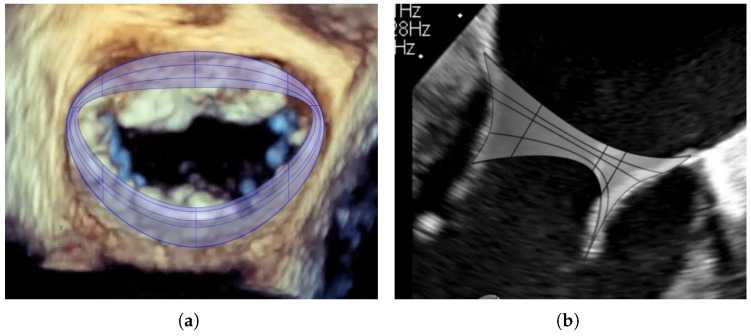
Comparison of the geometry at $t_{0}=0$ s with the ultrasound images. (a) Annulus geometry compared with a surgeon’s view ultrasound image. (b) Leaflet shape and annulus distance geometry compared with an ultrasound image.
